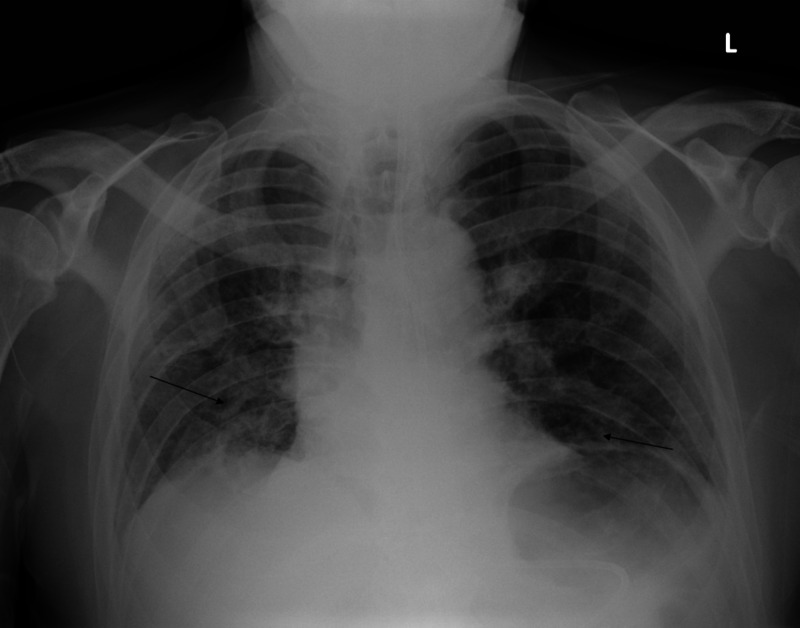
Chest X-ray revealing patchy bibasilar opacities with air bronchograms.
